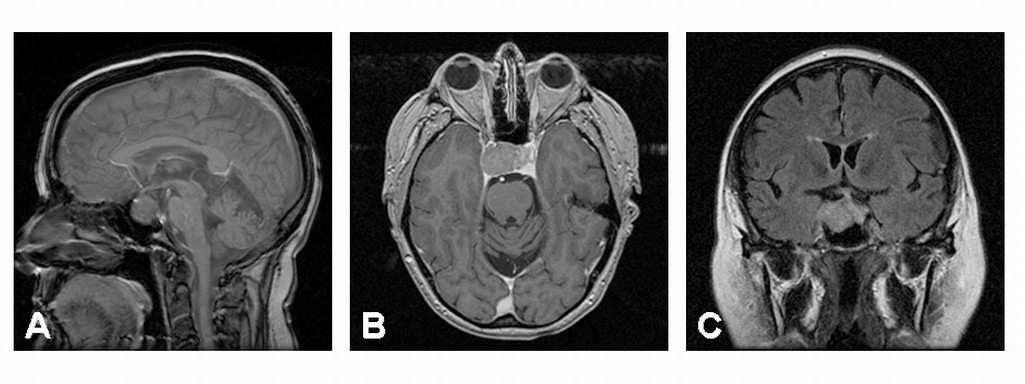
Pre-operative magnetic resonance imaging (MRI) of the head with and without contrast. Prior to endoscopic transnasal resection, midsagittal (A), axial (B) and coronal (C) MRI imaging reveal a homogeneous mass involving the sella turcica with extension into the right cavernous sinus which measures 2.8 cm × 2.1 cm × 1.7 cm. The mass does extend up into the suprasellar cistern, but does not impinge upon the optic apparatus. There is partial encasement of the cavernous internal carotid artery on the right side, but the caliber of the vessel is not compromised.
